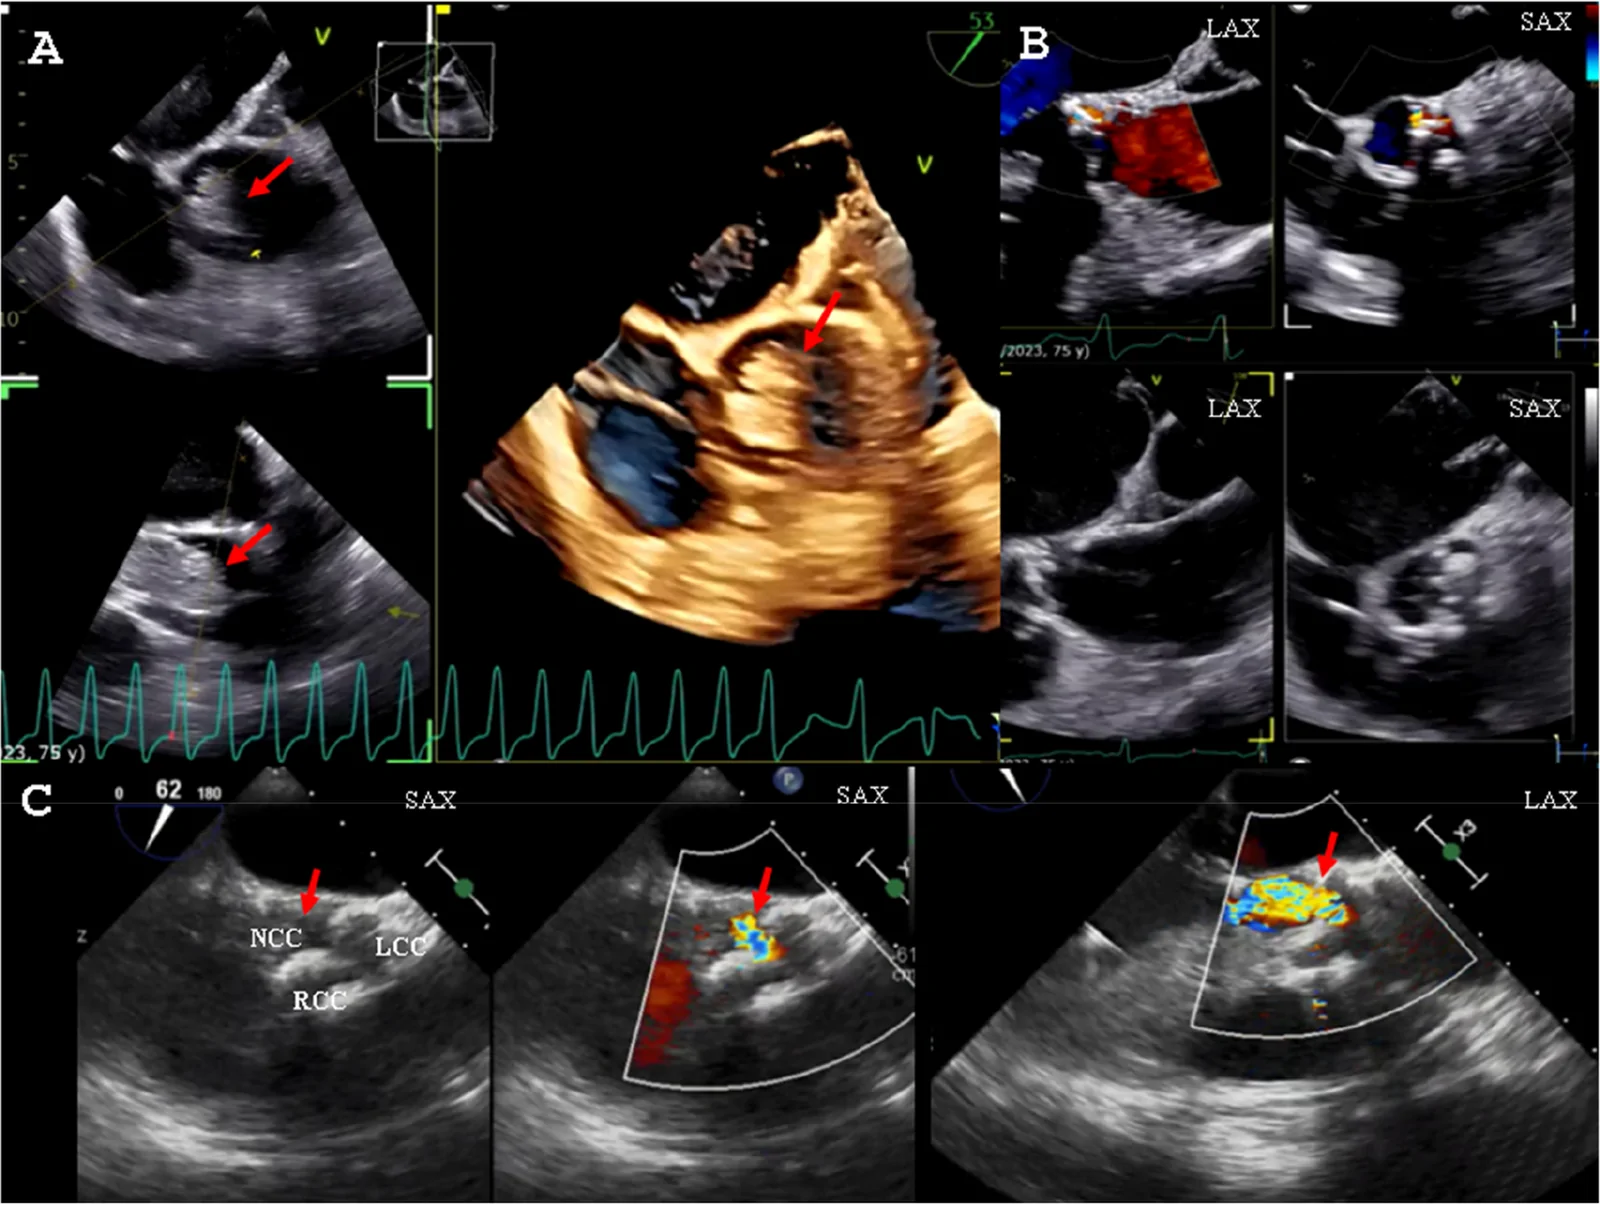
Balloon aortic valvuloplasty (BAV) in transcatheter aortic valve implantation (TAVI). A Real-time mini-transesophageal echocardiography (TEE) imaging of a severely calcified valve undergoing BAV. Red arrows point to the expanded balloon in 3D multiview (short axis [SAX], long axis [LAX], and 3D *en face* rendering views). B Mild aortic regurgitation (AR) was demonstrated post BAV. C In another example, acute severe AR occurred due to split noncoronary cusp (NCC) following BAV. Red arrows depict the split NCC commissure with resultant severe AR jet, that may cause acute hemodynamic compromise in patients with stiff and hypertrophied left ventricle. LCC, left coronary cusp; RCC, right coronary cusp
